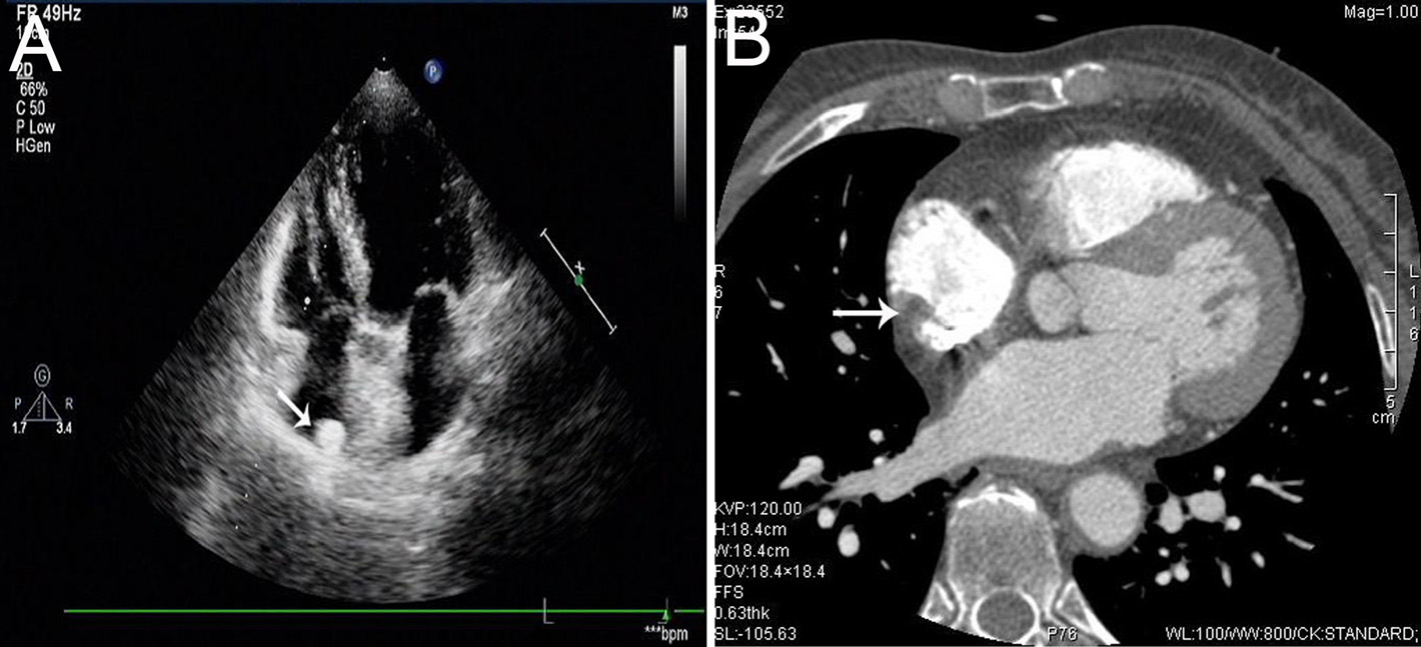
Echocardiogram and computed tomography. (A) Transthoracic echocardiogram showing a mass (arrow head) attached to the free wall of the right atrium. (B) A 64-multislice computed tomography (CT) showing a lipomatous mass (arrow head) on the free wall of the right atrium (arrow head).
